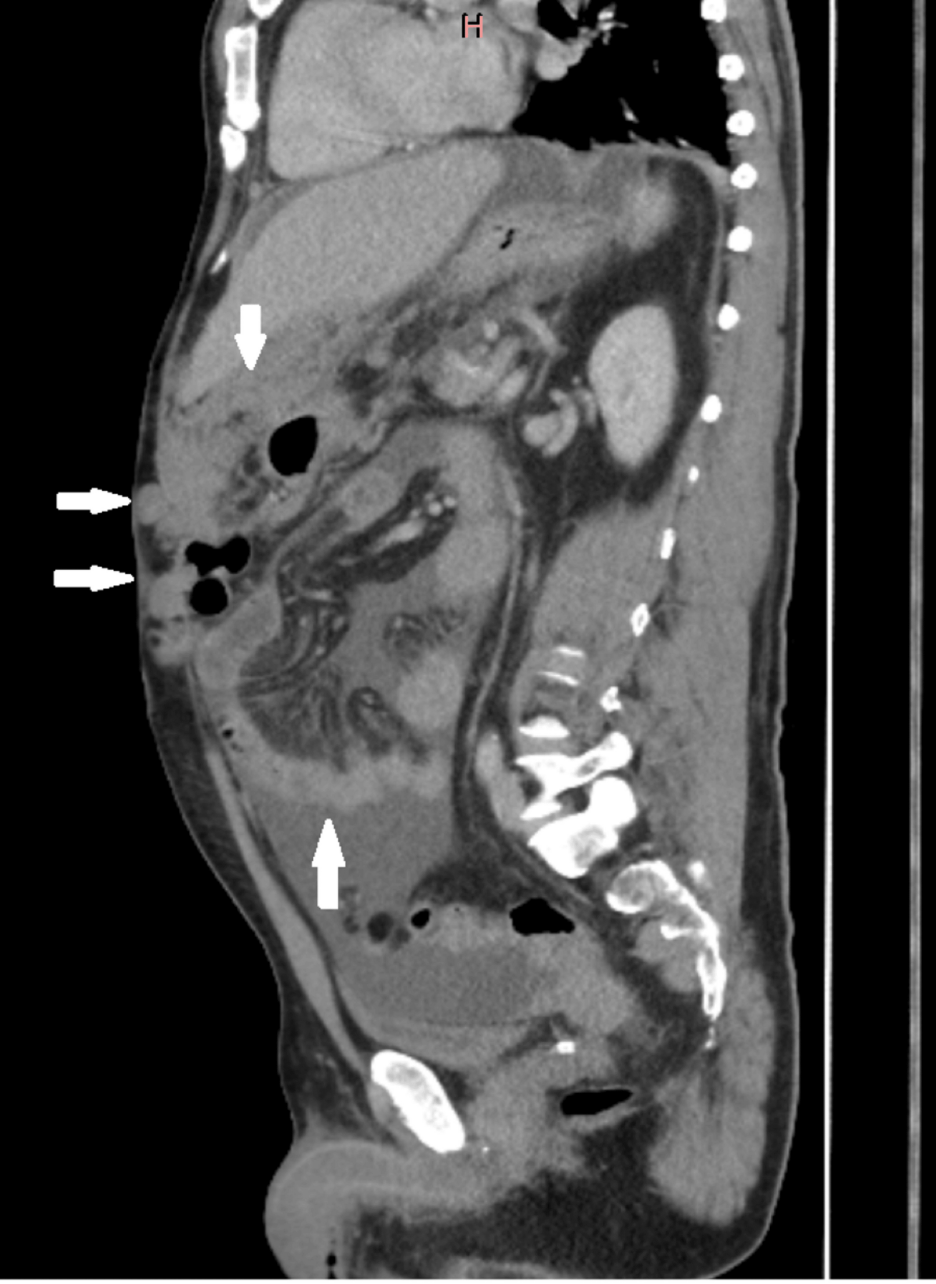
CT abdomen and pelvis (November 6, 2024): progression of peritoneal and omental deposits. The arrows indicate areas of omental and peritoneal deposits.
